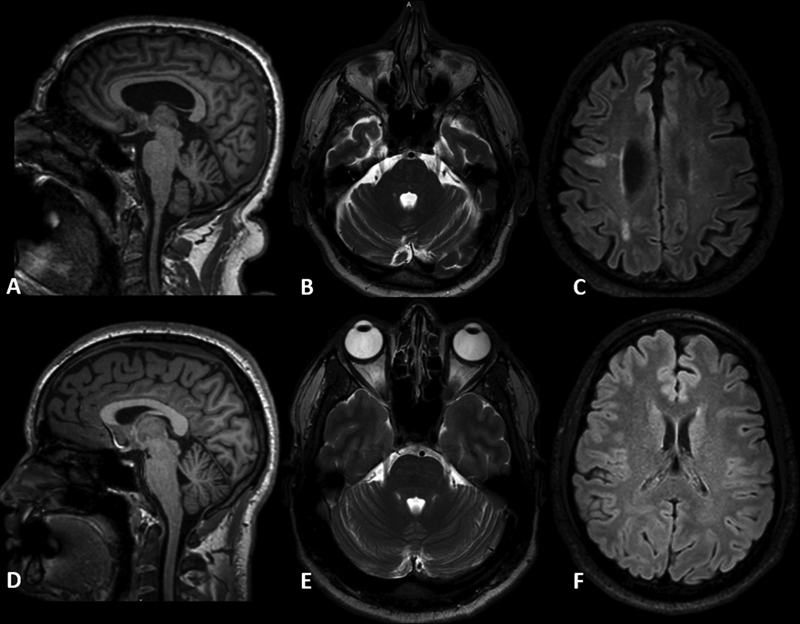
Sagittal and axial brain MRI scans of two CIDP patients. (
